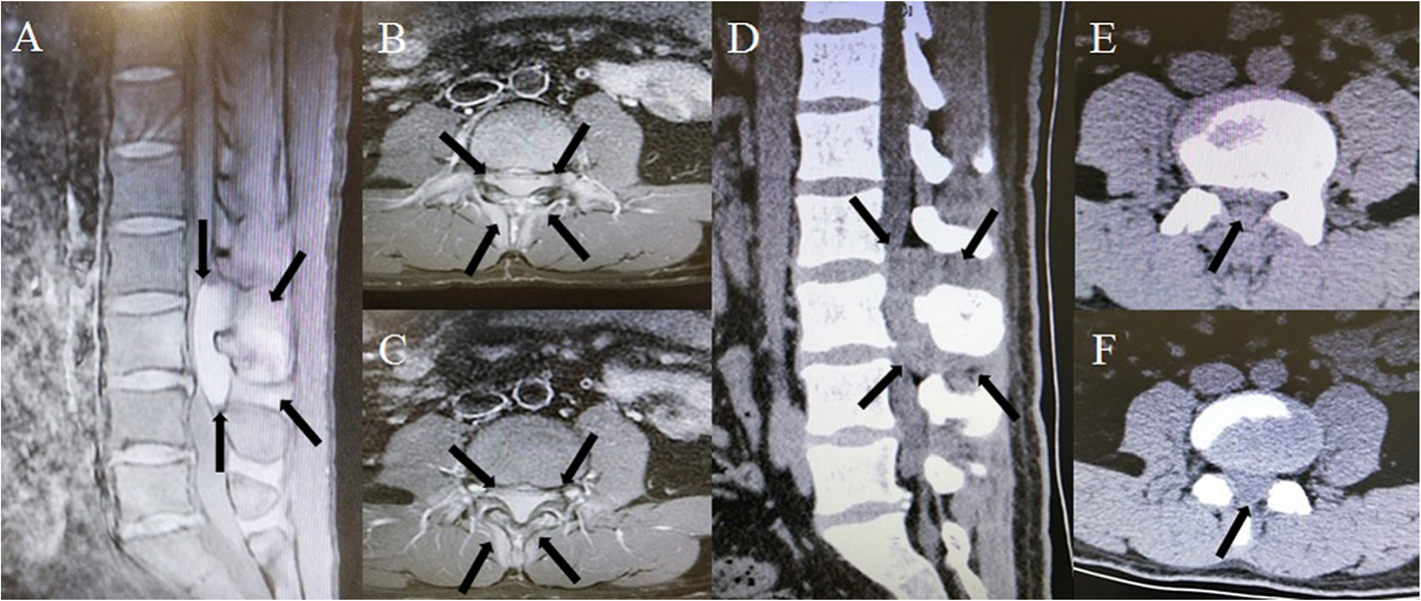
Preoperative enhanced T1-weighted magnetic resonance images (MRI) of the lumbar spine. a Sagittal and (b) (c) transverse views show an iso-intense, fusiform, high-signal lesion in the L2 to L4 vertebral canal, with complete compression of the local spinal cord and a mixed signal around the L3 lamina, spinous process, and soft tissue. Preoperative computed tomography (CT) of the lumbar spine. d Sagittal and (e) (f) transverse views show an iso-dense fusiform soft lesion at the L2 to L4 lumbar canal with local spinal cord compression and slight bone destruction.The black arrows show the scope of the lesion
